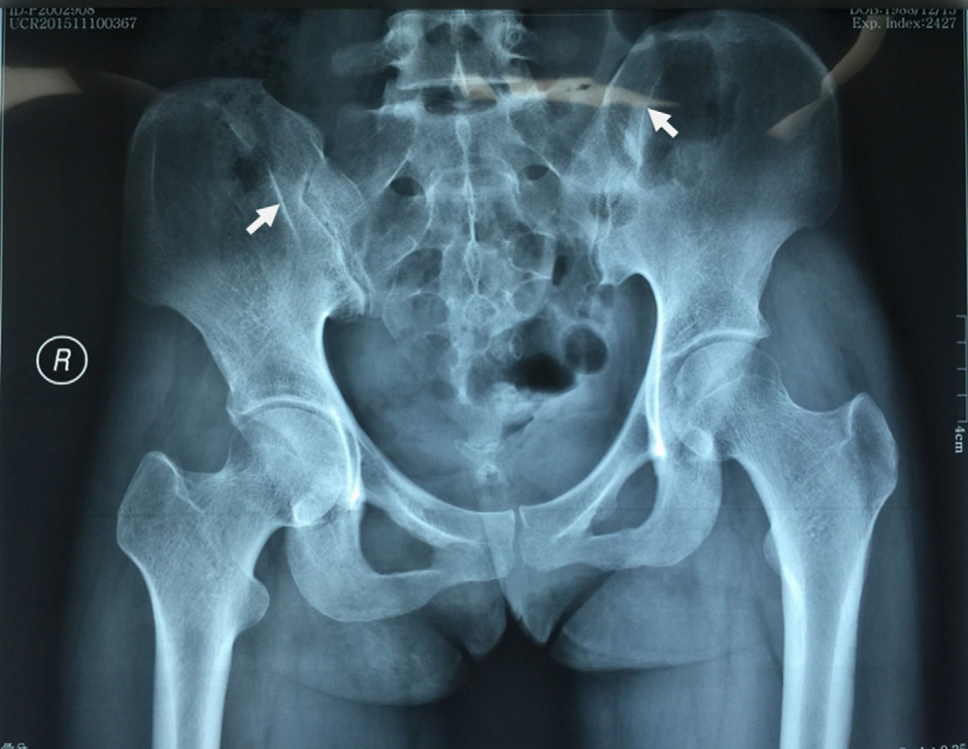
Anteroposterior radiograph of a patient with bilateral gluteal muscle contracture. The two arrowheads show iliac hyper-dense line over the bilateral posterior iliac spine with slight pelvic inclination toward the right.
